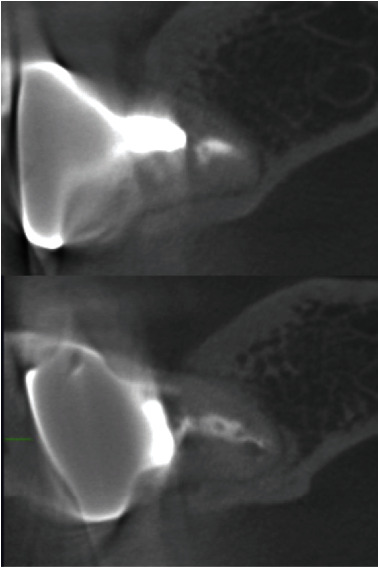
CBCT revealed the presence of buccal and lingual bone on both mesial (a) and distal (b) roots of the transplanted tooth.
